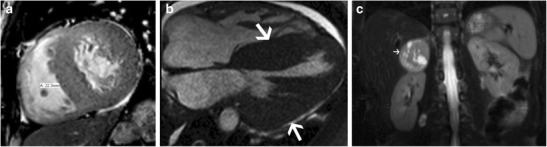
a Short axis cine-SSFP image in a patient with hypertension shows severe concentric LV hypertrophy with maximal thickness of 2.2 cm in the mid septum in end diastole. b 4-chamber cine SSFP image in another patient with HT shows severe concentric LV hypertrophy. c Coronal T1-weighted image through the upper abdomen after gadolinium administration shows an intensely enhancing mass in the upper abdomen (arrow), consistent with a pheochromocytoma
